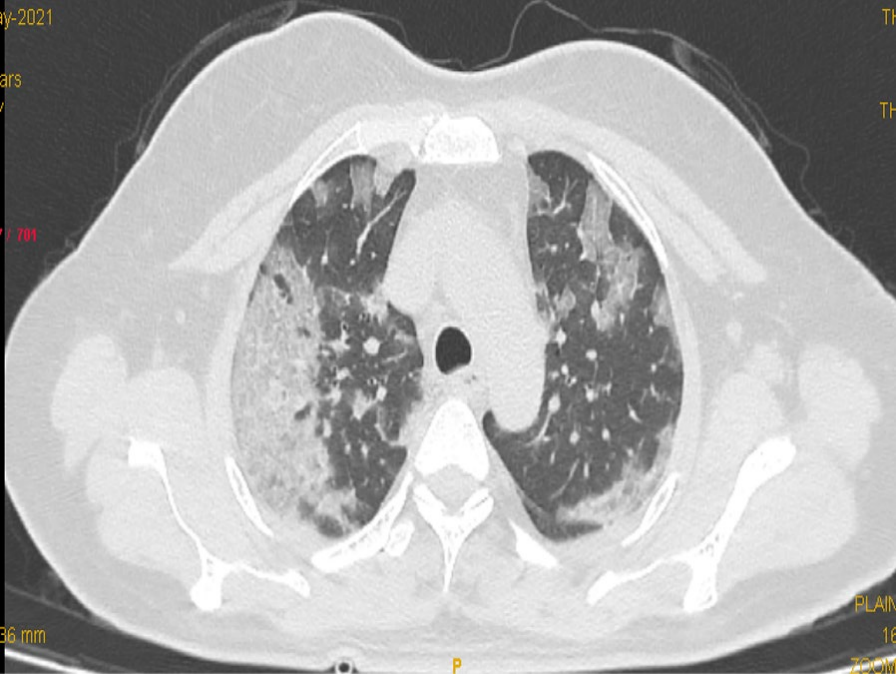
Axial CT image indicating moderate disease severity (CTSS 13). Axial CT image shows GGO with septal thickening and patchy consolidation in bilateral upper and lower lobes mainly in subpleural location with CTSS 13
